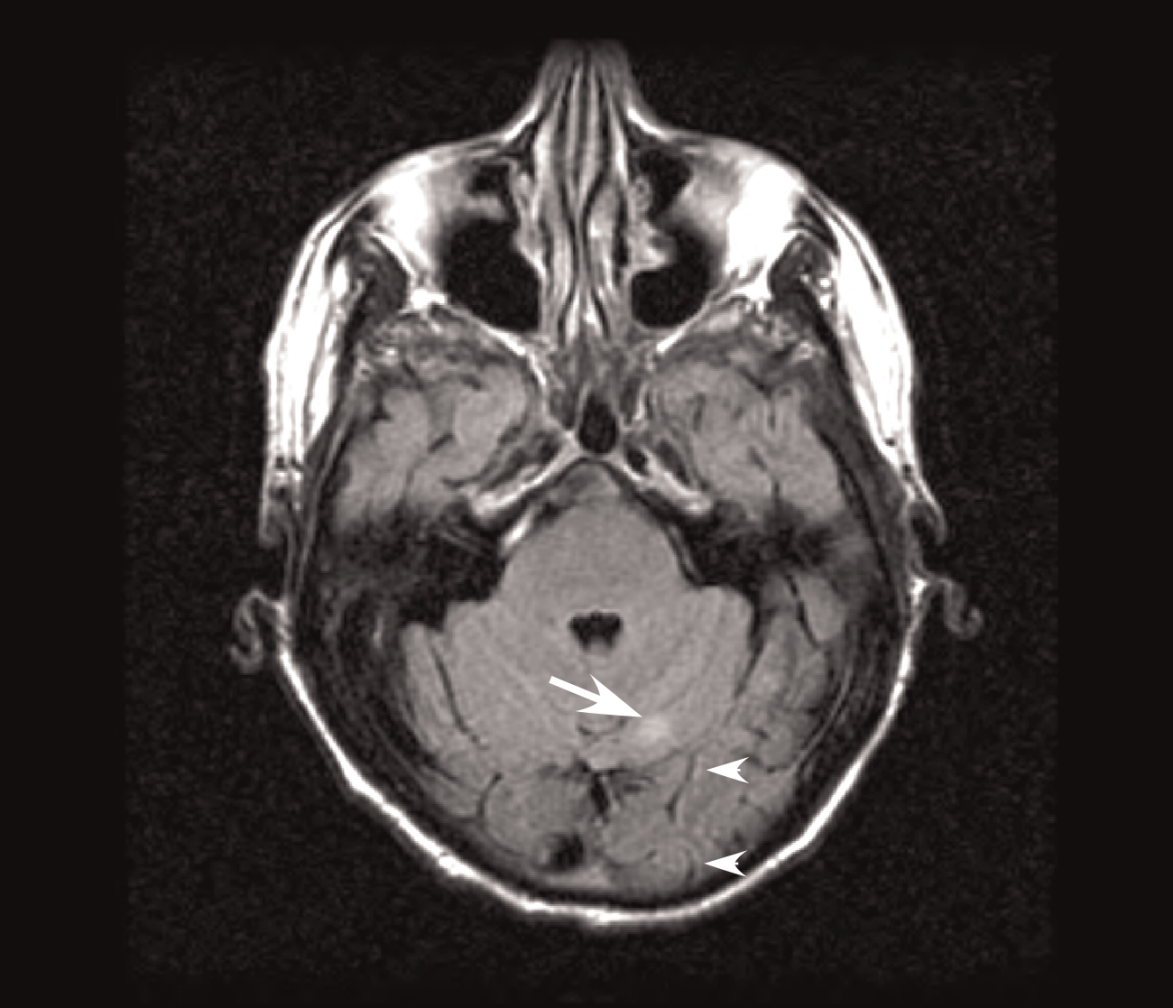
T1-weighted brain magnetic resonance imaging demonstrating both leptomeningeal enhancement (arrowheads) and a cerebellar metastasis (arrow).
